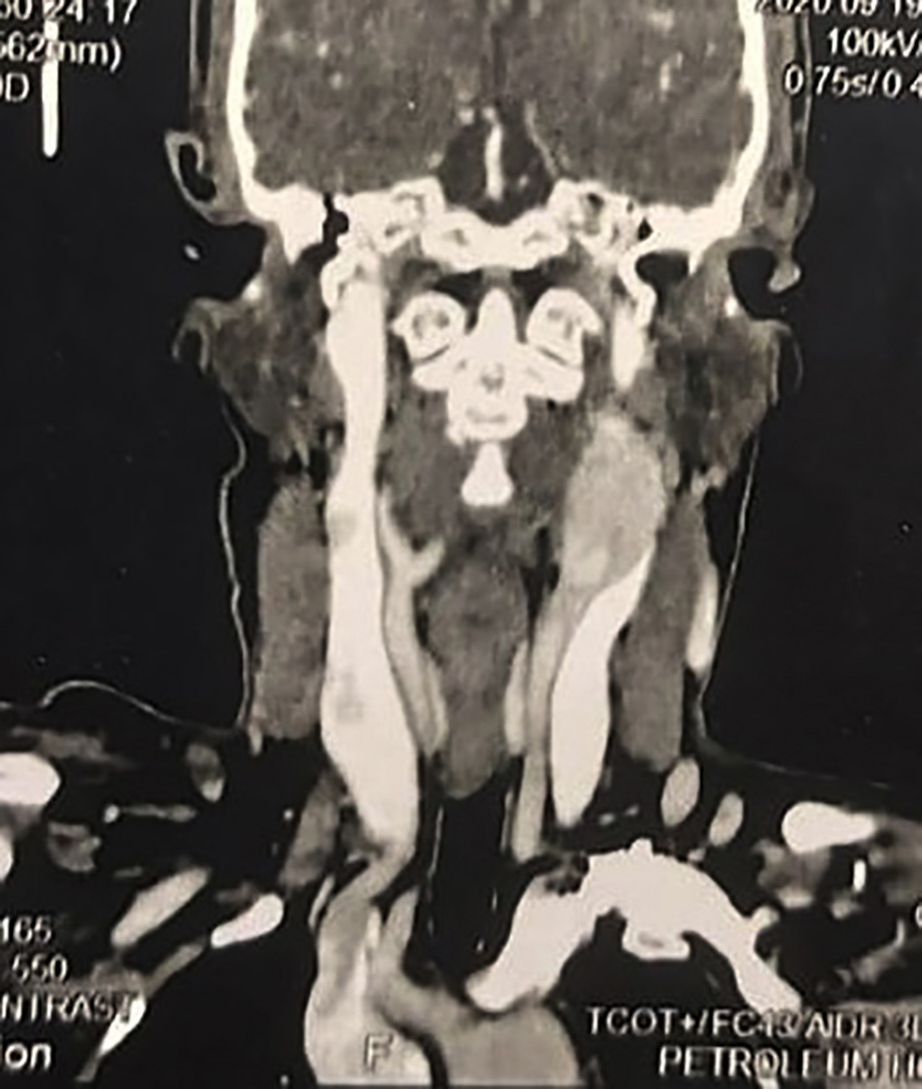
CT Angiography of Carotid Body Tumour Showing Vascular Involvement.
